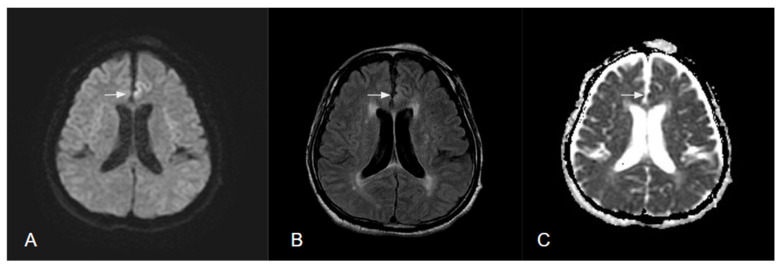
MR images of brain in a patient with status epilepticus. (A) Hyperintensity of DWI in cortical area and hypointensity in subcortical area. (B) Hyperintensity in T2 FLAIR at same region. (C) Hypointensity of ADC in cortical region.
